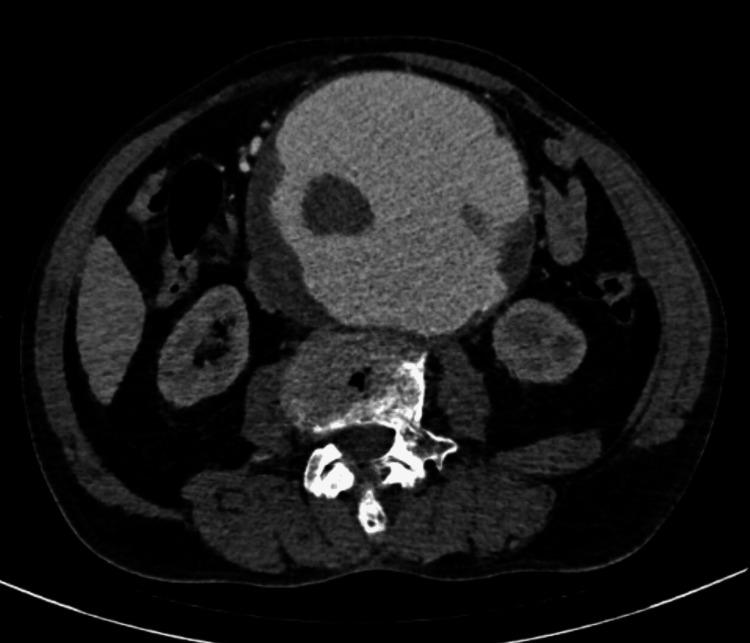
Contrast-enhanced axial CT of the abdomen Contrast-enhanced axial CT of the abdomen demonstrating a giant infrarenal AAA with extensive eccentric mural thrombus and a markedly reduced residual central lumen (true lumen measuring 86 mm in transverse diameter), features consistent with a high-risk aneurysm for imminent rupture. AAA, abdominal aortic aneurysm
